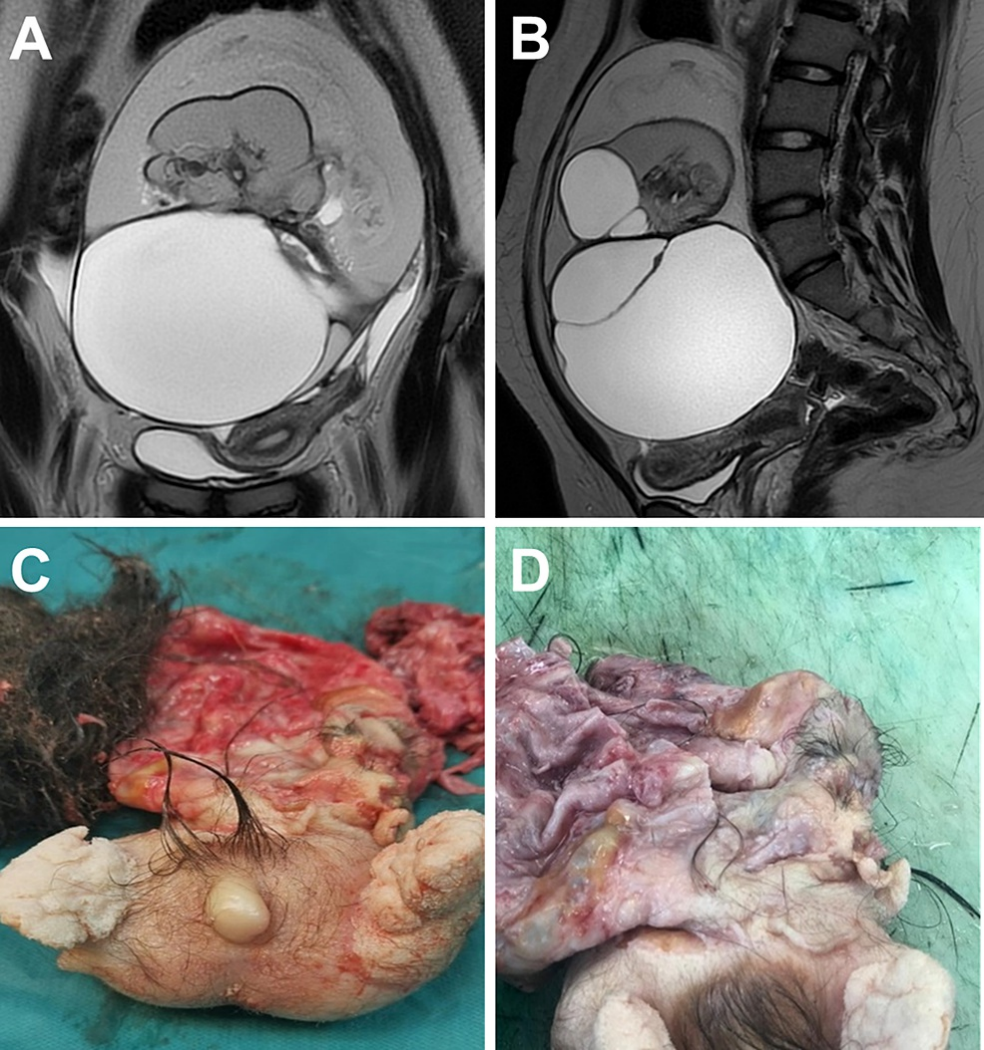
(A) Coronal and (B) sagittal magnetic resonance images showing a large left ovarian mass. (C) Gross morphology of the tumor showing two rudimentary limbs and a penis-like structure. (D) The tumor consisted of cystic (upper) and solid (lower) components.
